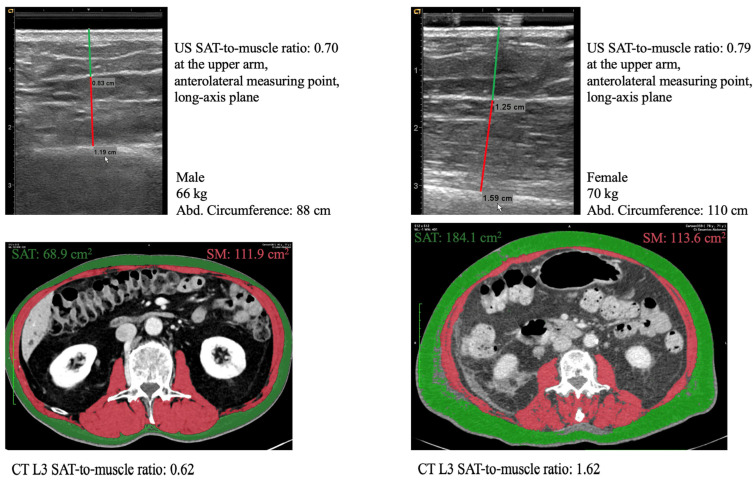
Ultrasound and CT SAT-to-muscle ratio in two representative patients. SAT: subcutaneous adipose tissue in green. SM: skeletal muscle in red. Please see Figure S6 for both the short- and long-axis planes to help distinguish SAT from muscle in the ultrasound scans.
